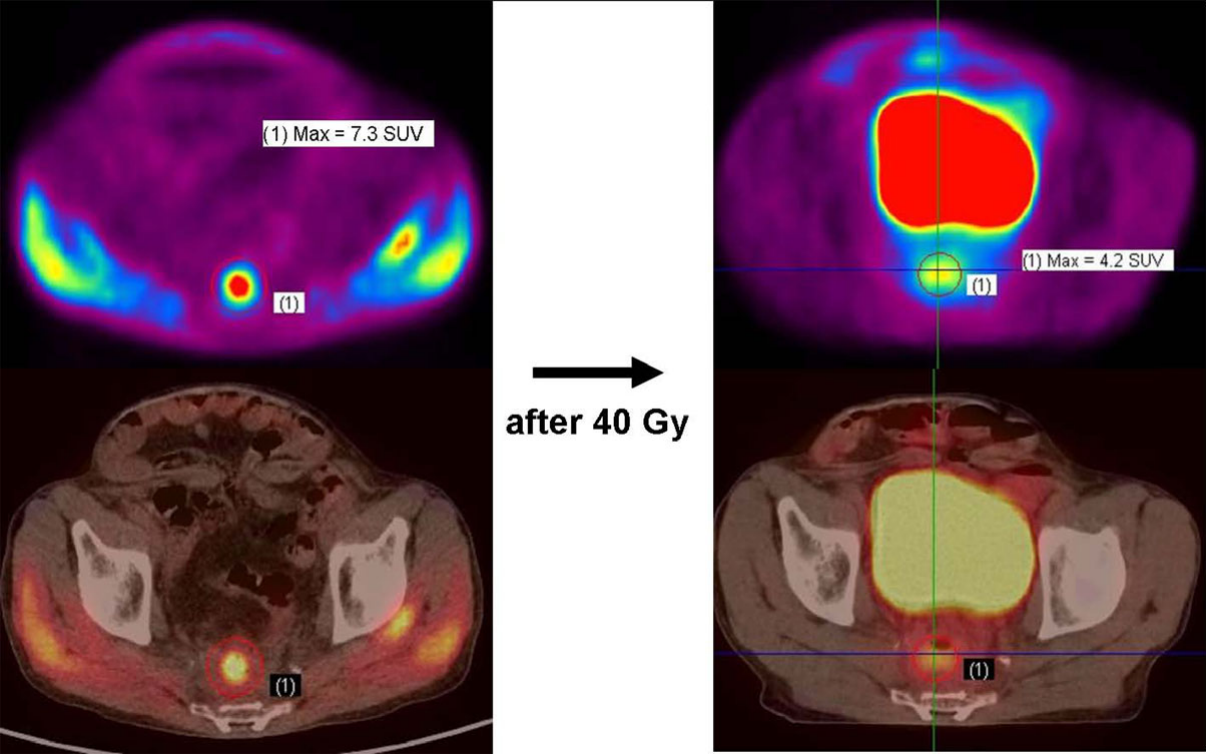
Change in FDG accumulation. This patient had anastomatic recurrence with 7.3 SUVmax before radiation therapy. After 40 Gy, the accumulation was decreased by 3.1 SUVmax.
